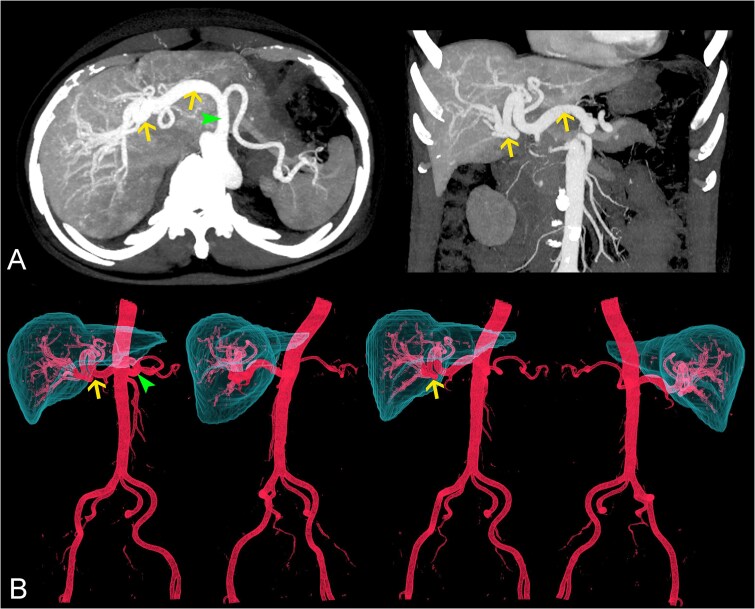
(A) Axial and coronal MIP and (B) 3D-reconstruction CT images showing diffuse dilatation of the celiac trunk (arrowheads) and hepatic arteries (arrows). Note the absence of abnormal shunts, signs of portal hypertension, masses, and aneurysms. The splenic artery showed normal size.
